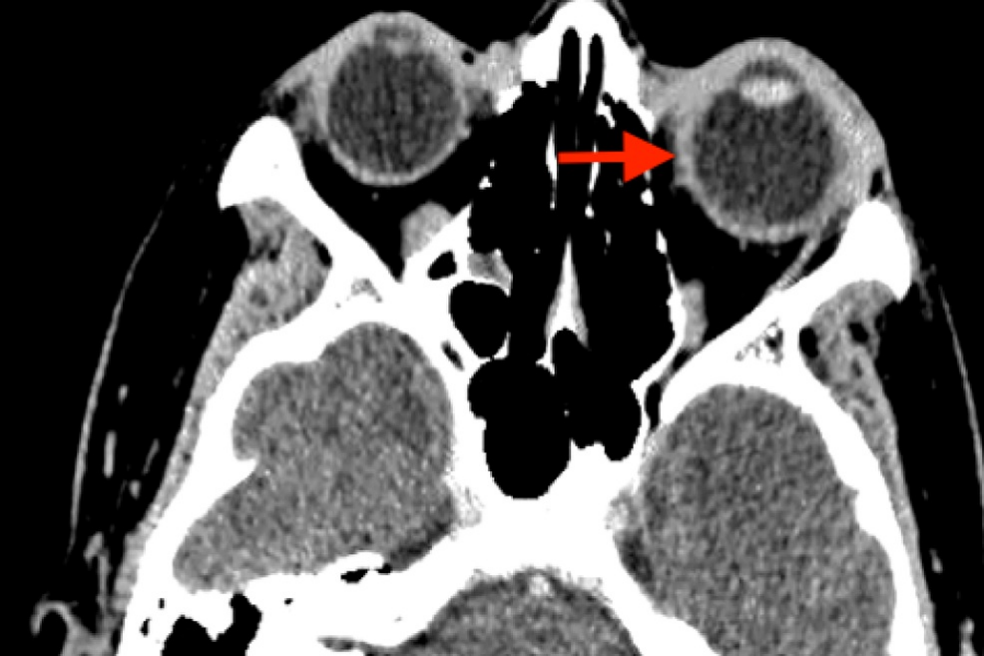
CECT brain/orbit shows left periorbital soft tissue thickening and enhancement suggestive of inflammatory/infective changes CECT: contrast-enhanced computed tomography
